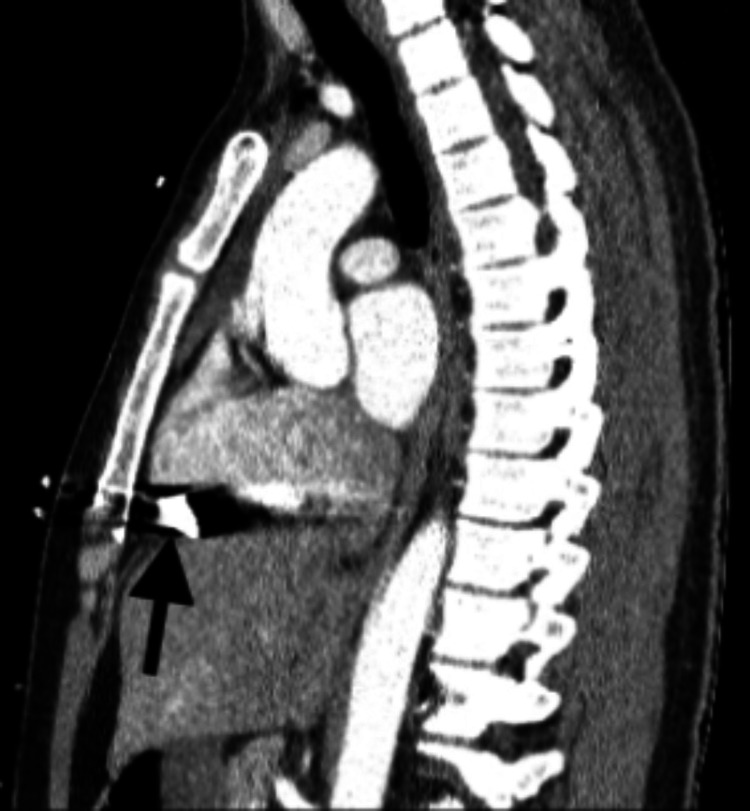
Sagittal CT chest demonstrates bullet lodged within the right ventricle of the heart. No evidence of pneumomediastinum, subcutaneous emphysema, fractures, or pulmonary lacerations to suggest the bullet directly entered the thoracic cavity.
